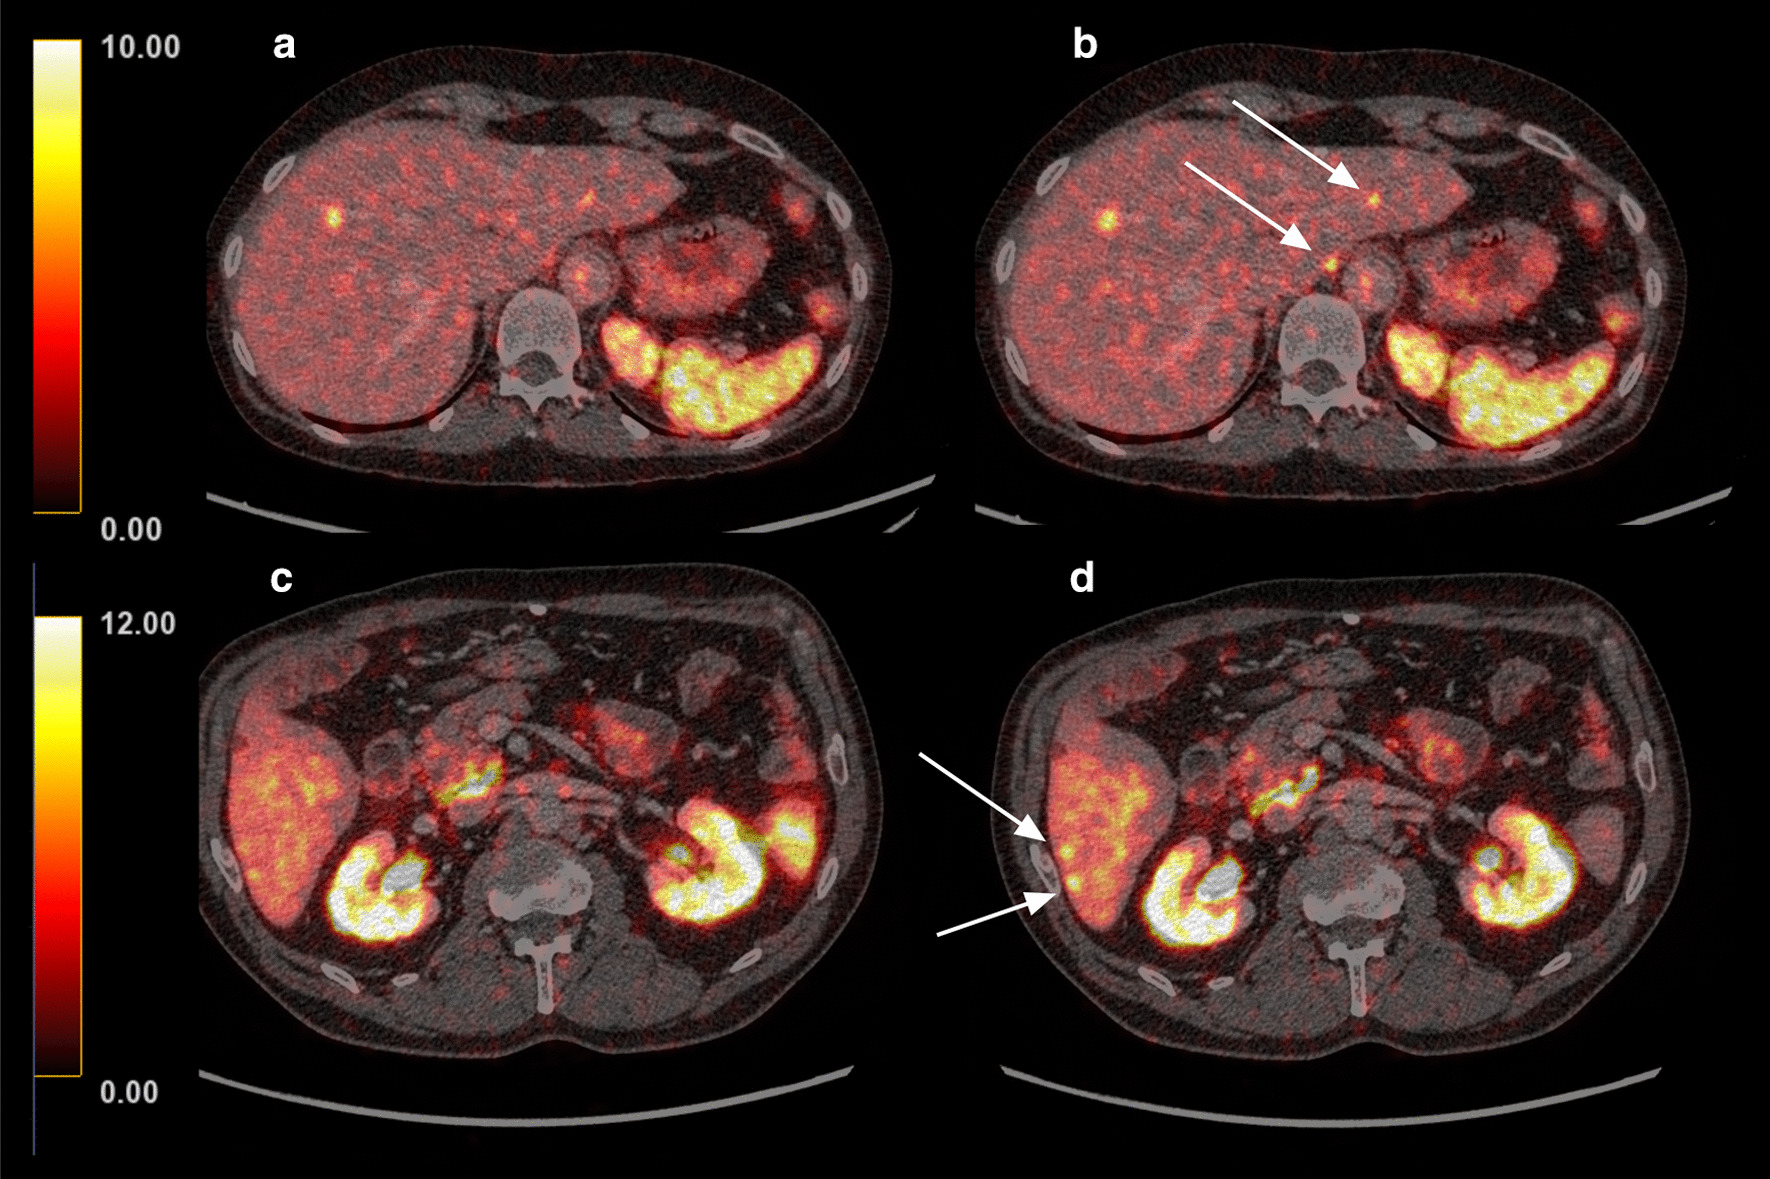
Fused [^68^Ga]Ga-DOTATOC PET/CT images in the transverse plane of two patients with small-intestinal neuroendocrine tumours in the upper panel (a, b) and in the lower panel (c, d), respectively. Non-gated images are shown to the left (a, c) and the gated images to the right (b, d). In each patient, two additional lesions were diagnosed in the gated image (indicated by arrows)
